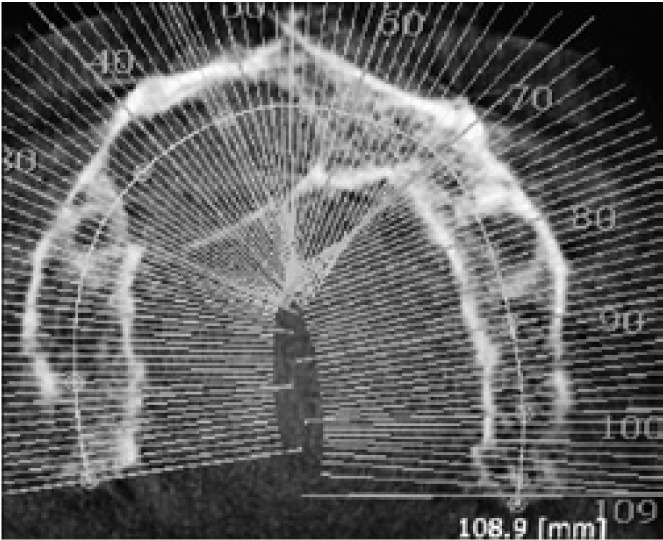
CBCT showing the borders of a KCOT. Note that the lesion shows cortical expansion at the both of the vestibular and palatal side of the maxilla.
